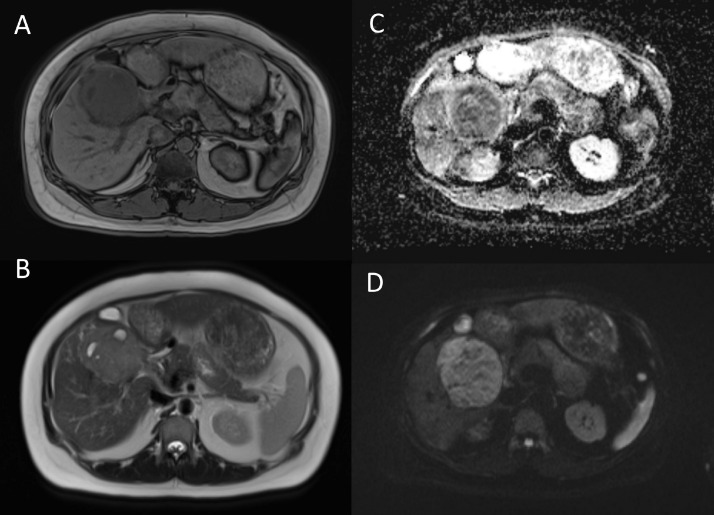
MRI images showing a large well-defined exophytic lesion at segment V/VI 7.1 × 7.4 × 7.8 cm with few cystic components seen within, and a clear fat plane with surrounding structures. On T1WI it has heterogenous hypointense signal (A) and on T2WI a hyperintense signal (B). No signal loss in out-of phase sequence. Evidence of restricted diffusion seen showing high signal on DWI (C) and low signal on ADC sequence (D).
